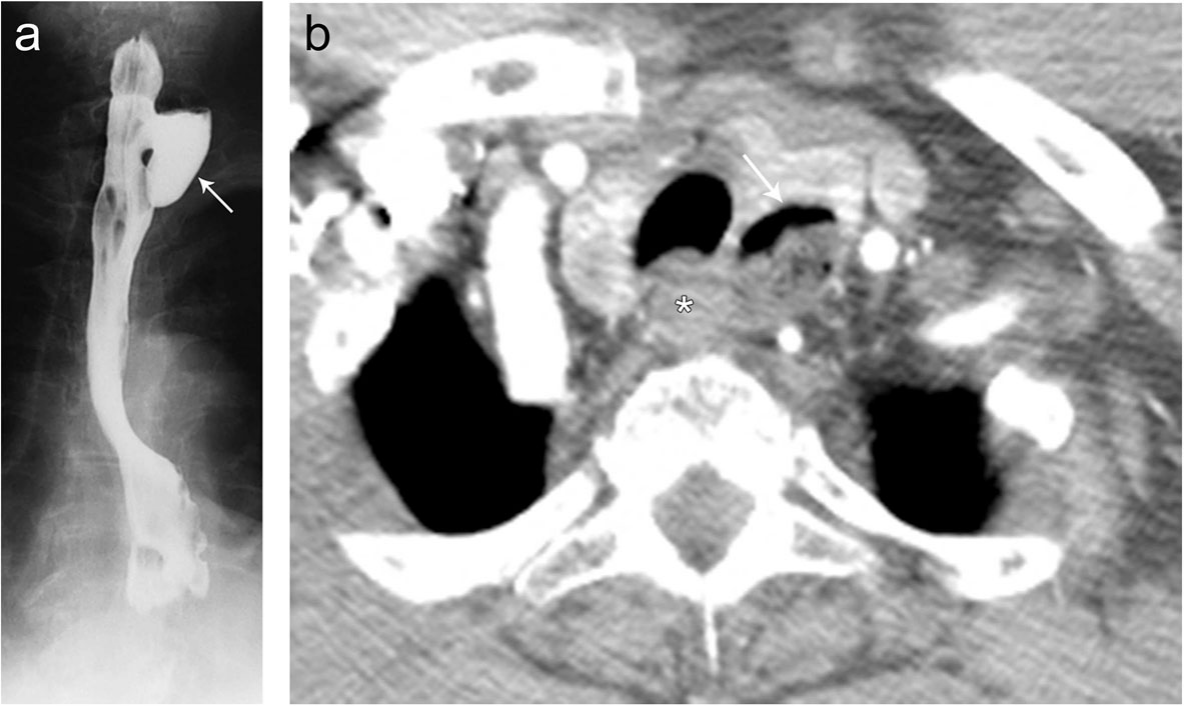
Killian-Jamieson diverticulum. Frontal (a) fluoroscopic images from an esophagram demonstrate a focal outpouching of the anterolateral aspect of the esophagus containing an air-fluid level consistent with a Killian-Jamieson diverticulum. These lesions are rarely bilateral and are typically smaller than a Zenker diverticulum (< 1.5 cm). Coronal (b) CT image from the same patient demonstrates air and oral contents (arrow) within the Killian-Jamieson diverticulum arising from the anterolateral aspect of the esophagus (asterisk)
